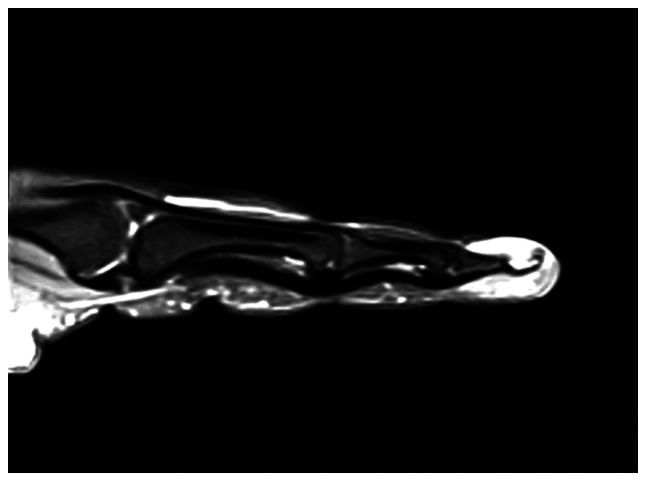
Sagittal proton density-weighted magnetic resonance imaging scan of the lesion.
